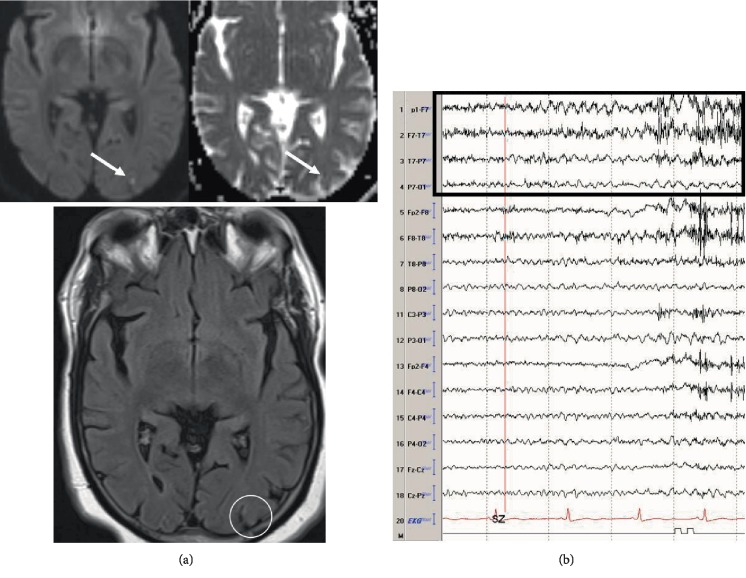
(a) MRI of the brain showing restricted diffusion on diffusion weighted imaging and corresponding decrease on apparent diffusion coefficient sequence (arrows, top images) and subtle hyperintensity on fluid attenuated inversion recovery sequence (circle, bottom image). (b) Bitemporal montage (5 second epoch, 50 *μ*v, sn 7 *μ*V/mm; LFF 1 Hz, HFF 70 Hz) showing a left temporal seizure (black box).
